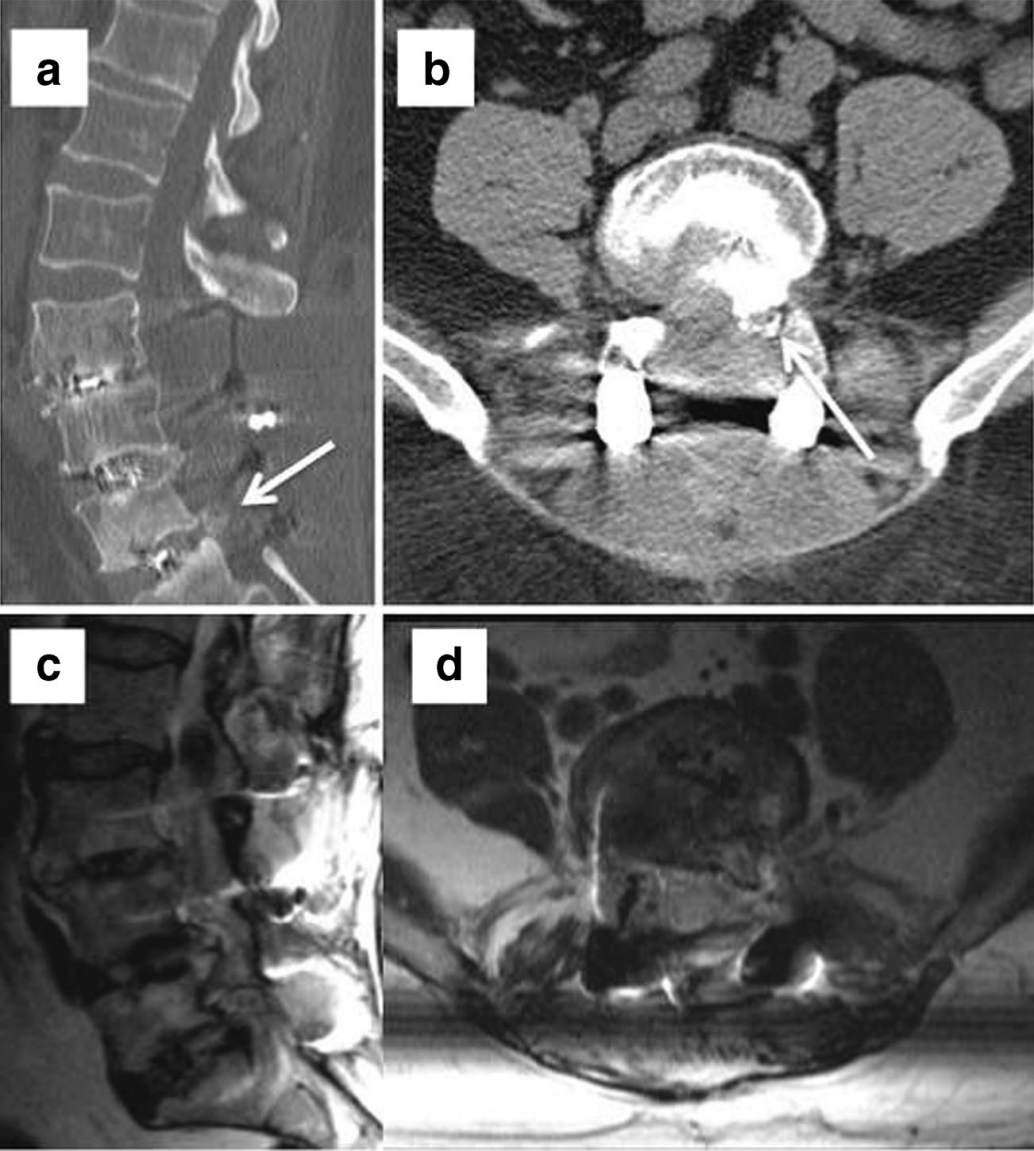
Heterotopic bone formation along the left ventrolateral aspect of the canal with left lateral recess stenosis is seen on CT (a and b) as well as sagittal and axial T2 WI (c and d)
